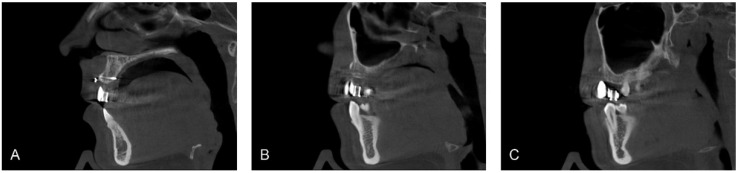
Cone-beam CT-Scan with radiographic stent *in situ* at six months after bone augmentation. Sagittal CT-images representing region 11 (A); 13 (B) and 14 (C). Sufficient vertical and horizontal bone volume in region 11 and 13 (A,B), reduced horizontal bone volume in region 14 (C). Images: Institute of Radiology, Kantonsspital Aarau, AG, Aarau, Switzerland.
